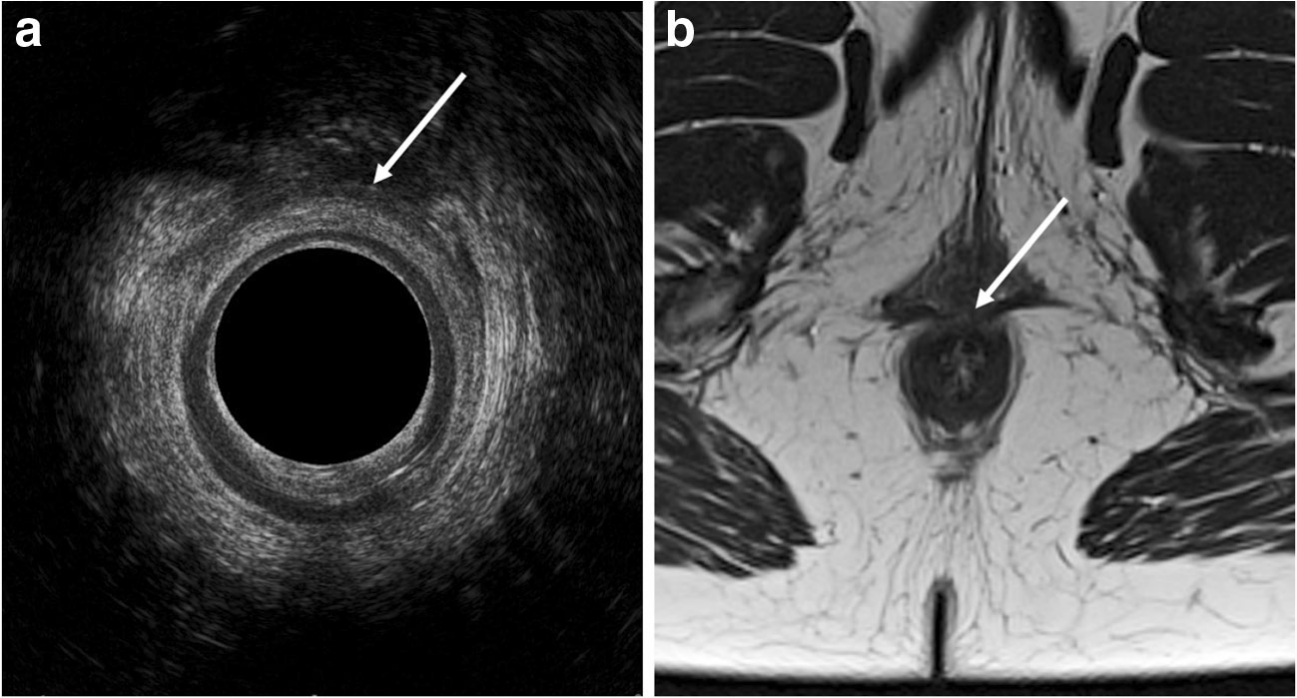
Image of an anterior EAS defect. a EAUS image. b MR image. The defect is marked with a white arrow
